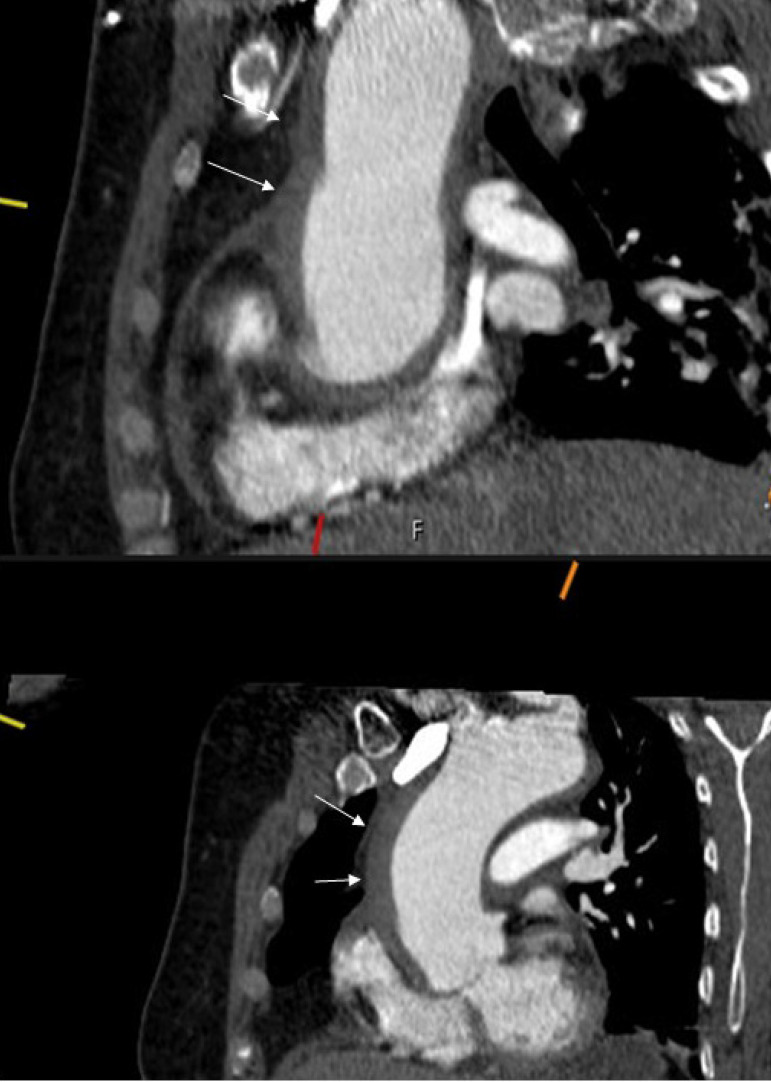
Computed tomography angiographic images showing type A intramural
